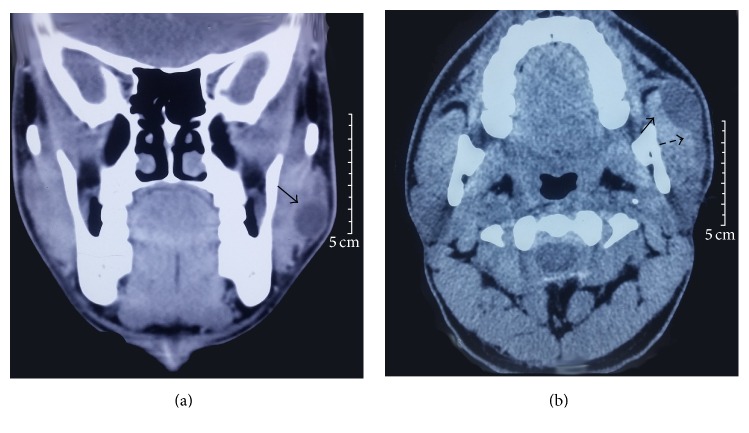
Coronal (a) and axial (b) cuts of computed tomography scan showing a small 2.2 cm × 1.5 cm, well defined, hypodense cystic lesion superficial to the anterolateral aspect of the left masseter muscle indenting its surface (solid arrow), communicating with a tubular hypodense structure (dashed arrow) communicating with the parotid gland, suggestive of a dilated Stensen's duct with a sialocele formation.
